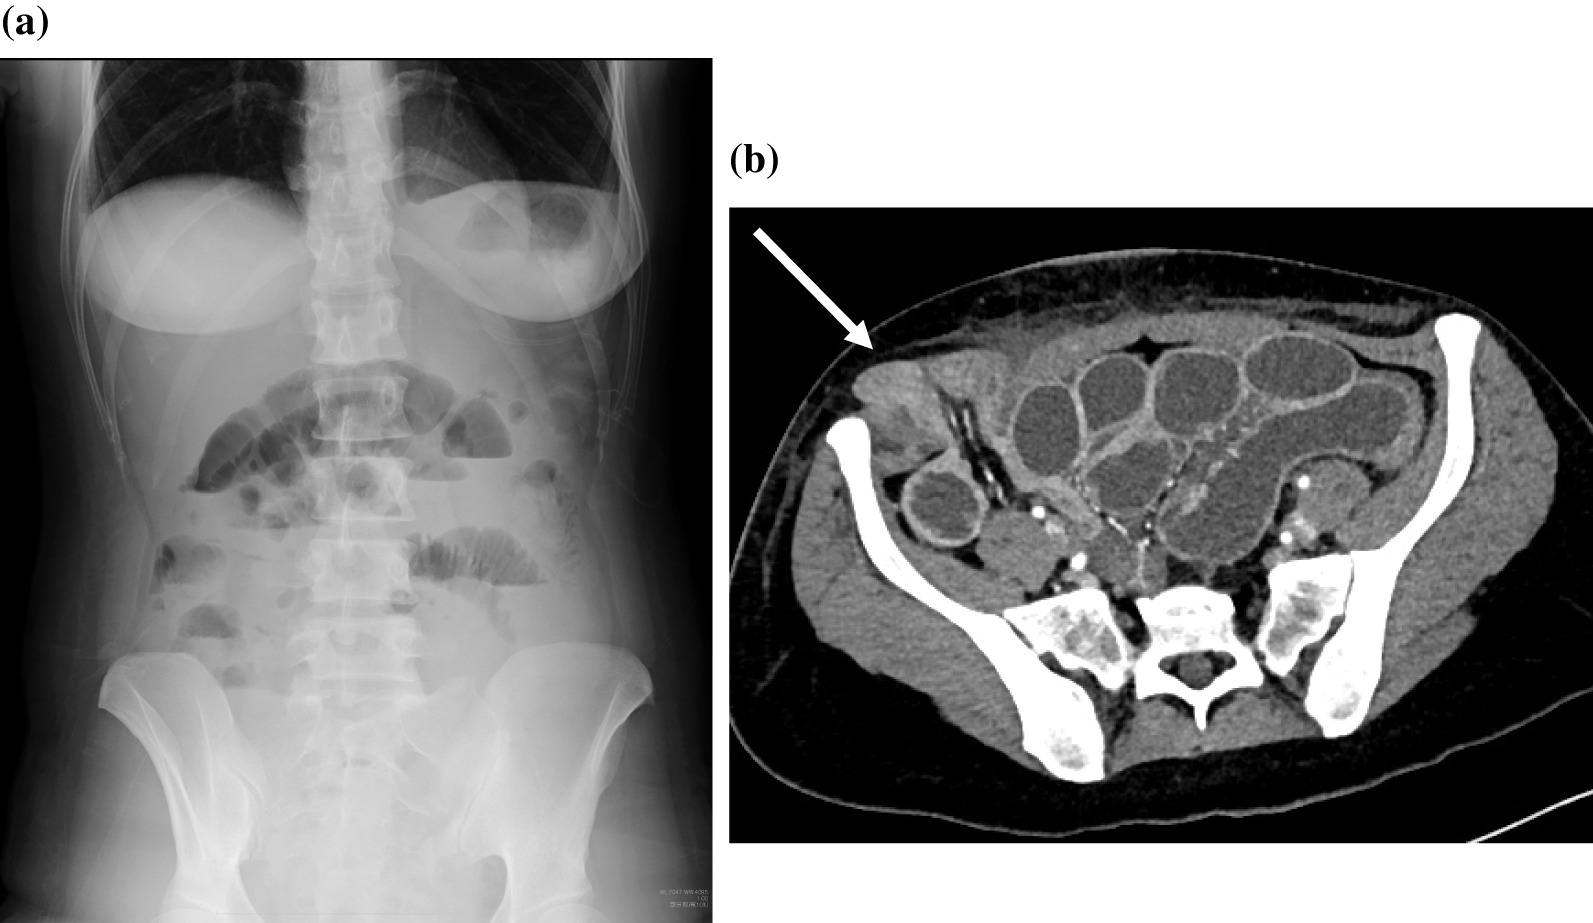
a The abdominal X-ray demonstrated multiple air-fluid levels, suggesting small bowel obstruction. b The abdominal contrast enhanced computed tomography scan of the small bowel loops above the fascia in the patient with herniation through a 12-mm left lateral trocar site (arrow)
